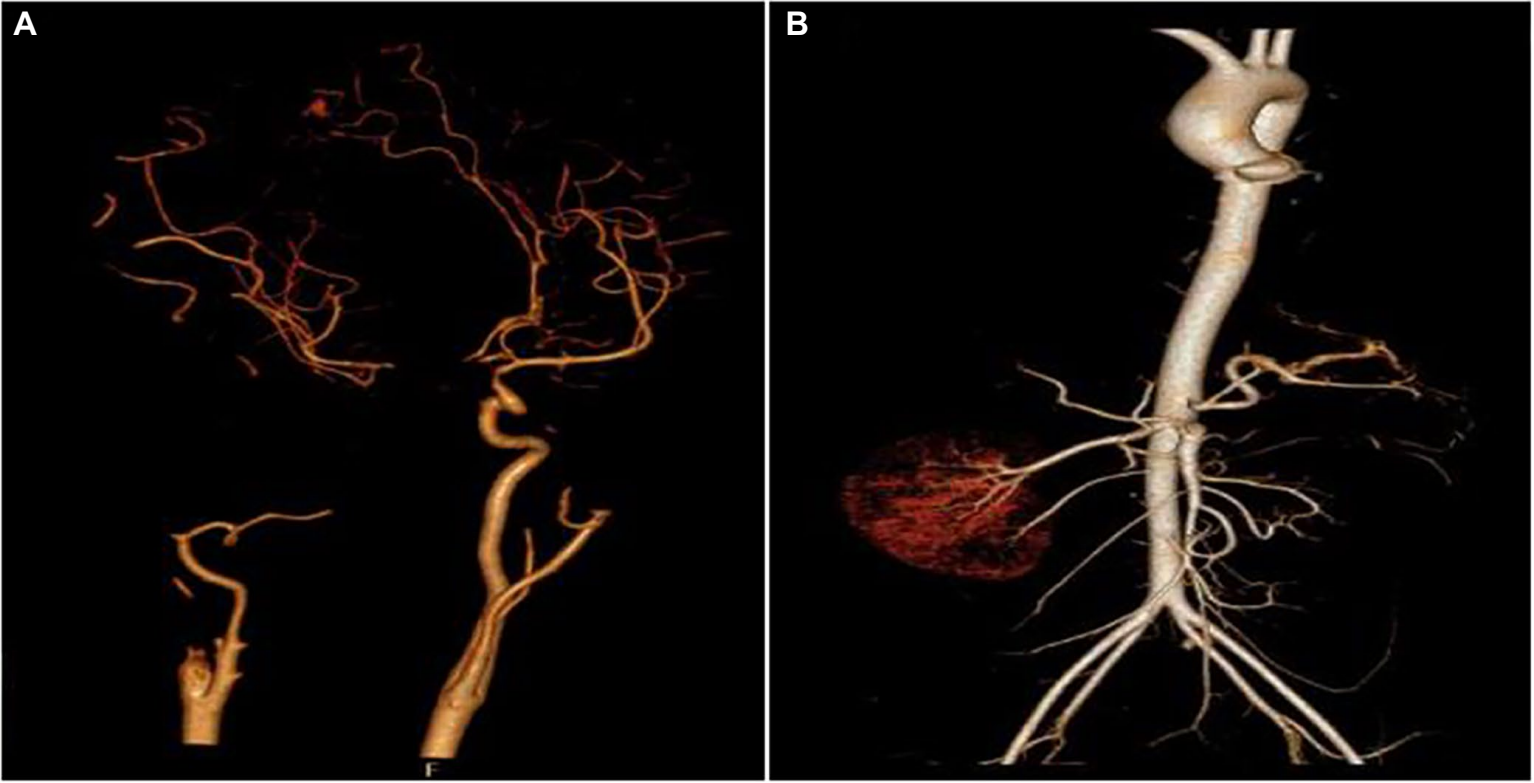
Aortic computed tomography angiography (CTA) of the cranial and abdominal artery 70 days after admission. (A) Right internal carotid artery and M1 middle cerebral artery were absent and (B) the left kidney and the left renal artery were absent.
